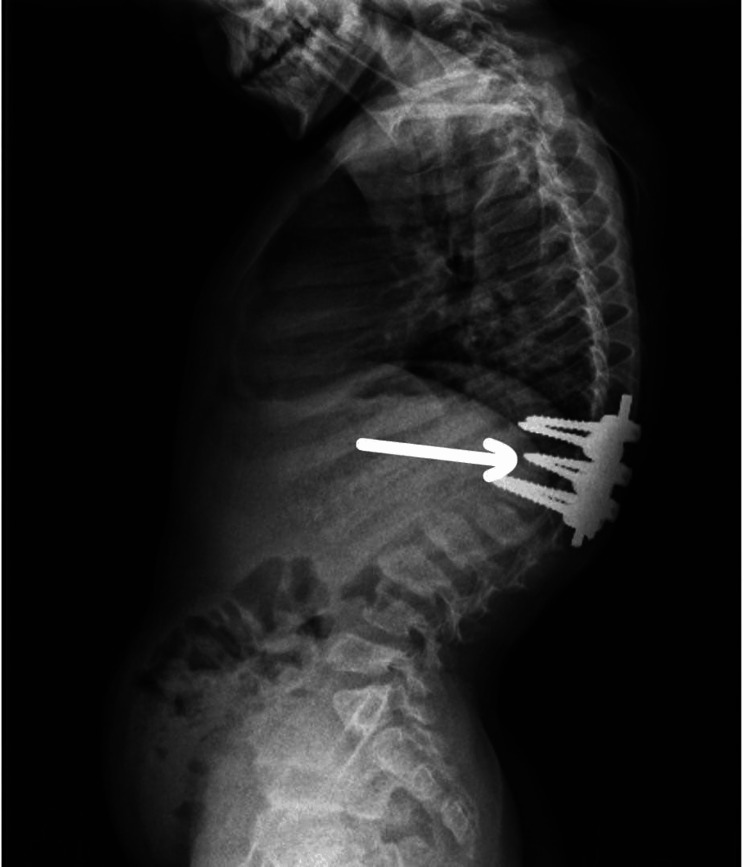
Lateral X-ray view: Postoperative image demonstrating spinal fixation in a patient with Morquio syndrome, showing severe kyphosis and vertebral abnormalities characteristic of the disorder.
